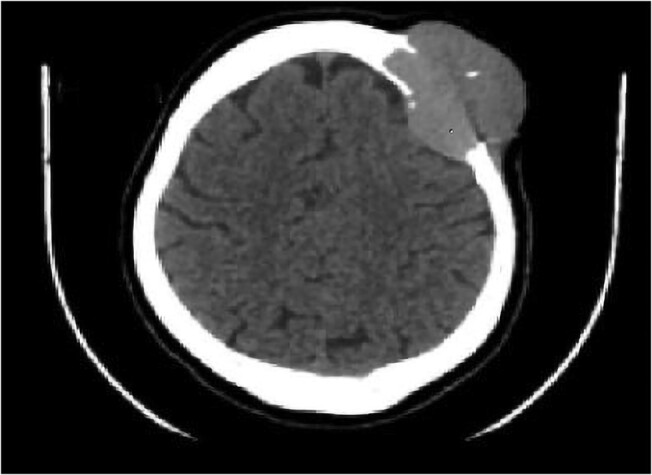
Axial image on MRI showing left frontal bone mass that is well-circumscribed with intra- and extra-cranial component and mass effect on the left frontal lobe.
